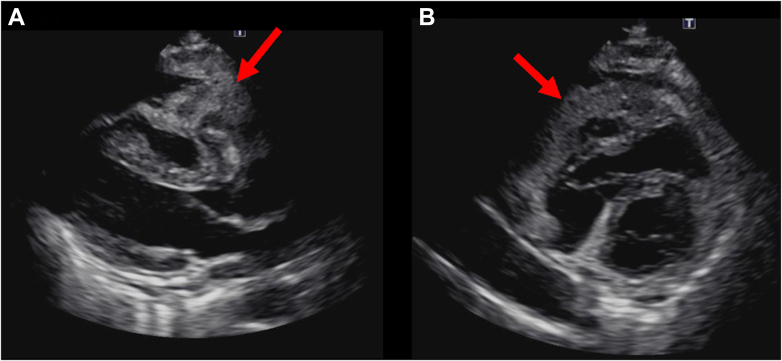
Initial Transthoracic Echocardiography Imaging reveals a mass attached to the right atrium and right ventricle (red arrows) and pericardial effusion in the pericardial cavity. (A) Parasternal long-axis view and (B) parasternal long-axis view.
